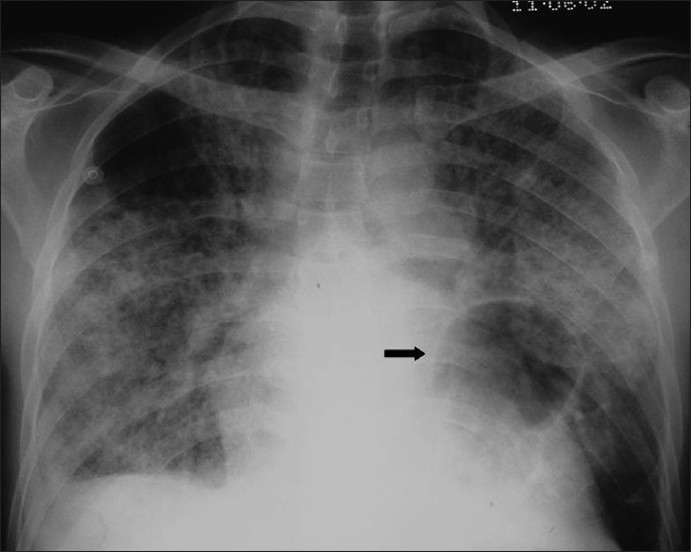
Pneumocystis carinii* pneumonia. If left untreated, chest X-ray may progress to alveolar consolidation in 3 or 4 days. Infiltrates clear within 2 weeks, but in a proportion infection will be followed by coarse reticular opacification and fibrosis. Note the large cyst (arrow)
